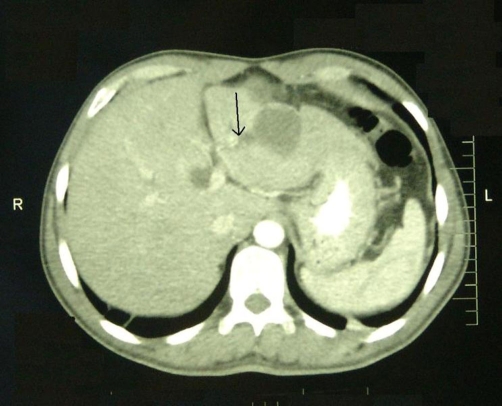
CT of the abdomen revealing a polychorous cyst in the left hepatic lobe with rupture in the biliary tract (arrow).
